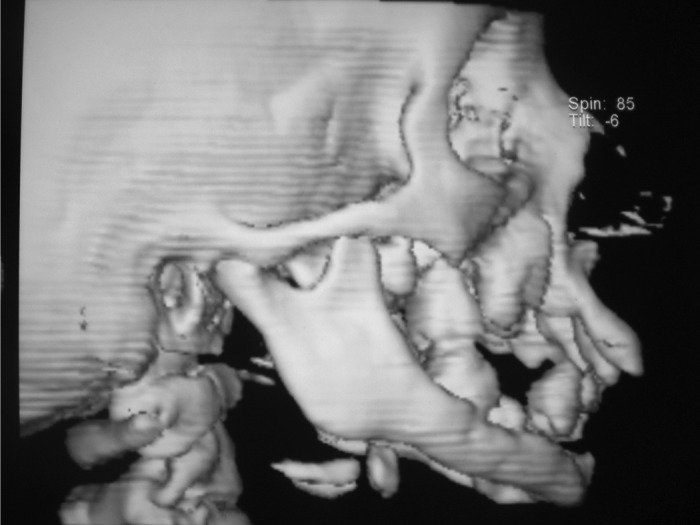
3D reconstructive view right side of CT-face
